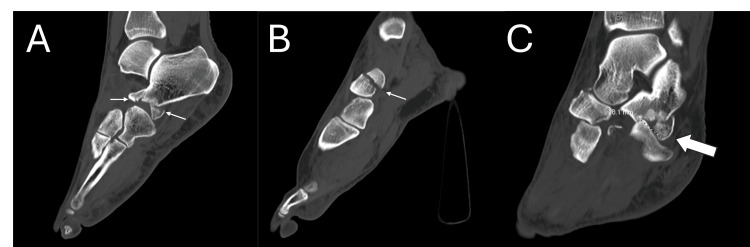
Selected sagittal (A, B) and coronal (C) cuts from preoperative CT imaging demonstrating naviculocuneiform dislocation with navicular fracture (small arrow (B)) and calcaneocuboid dislocation with fractures of the anterior process of the calcaneus (small arrows (A); large arrow (C)).
